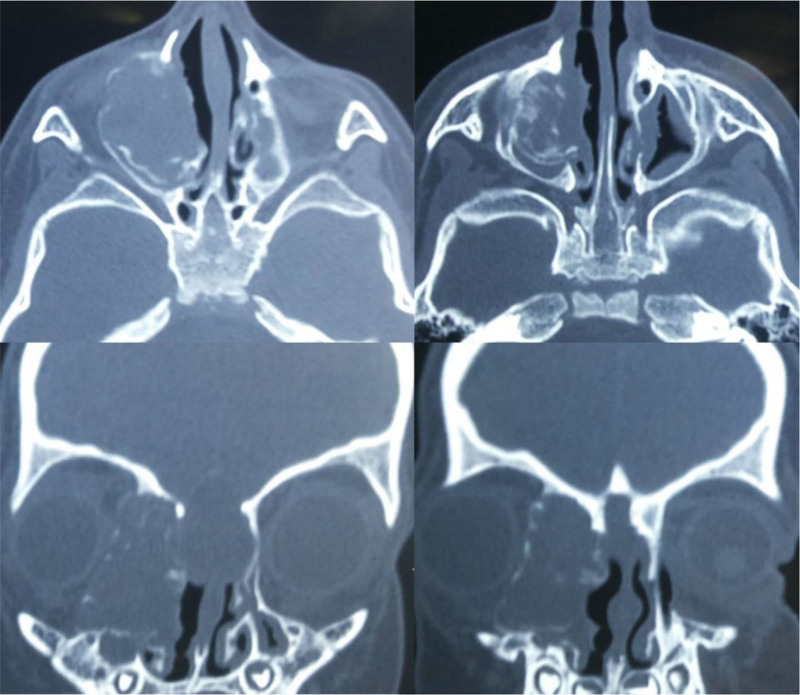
Non-contrast computed tomography revealed an extradural mass extending into the periorbital region and anterior skull base.
